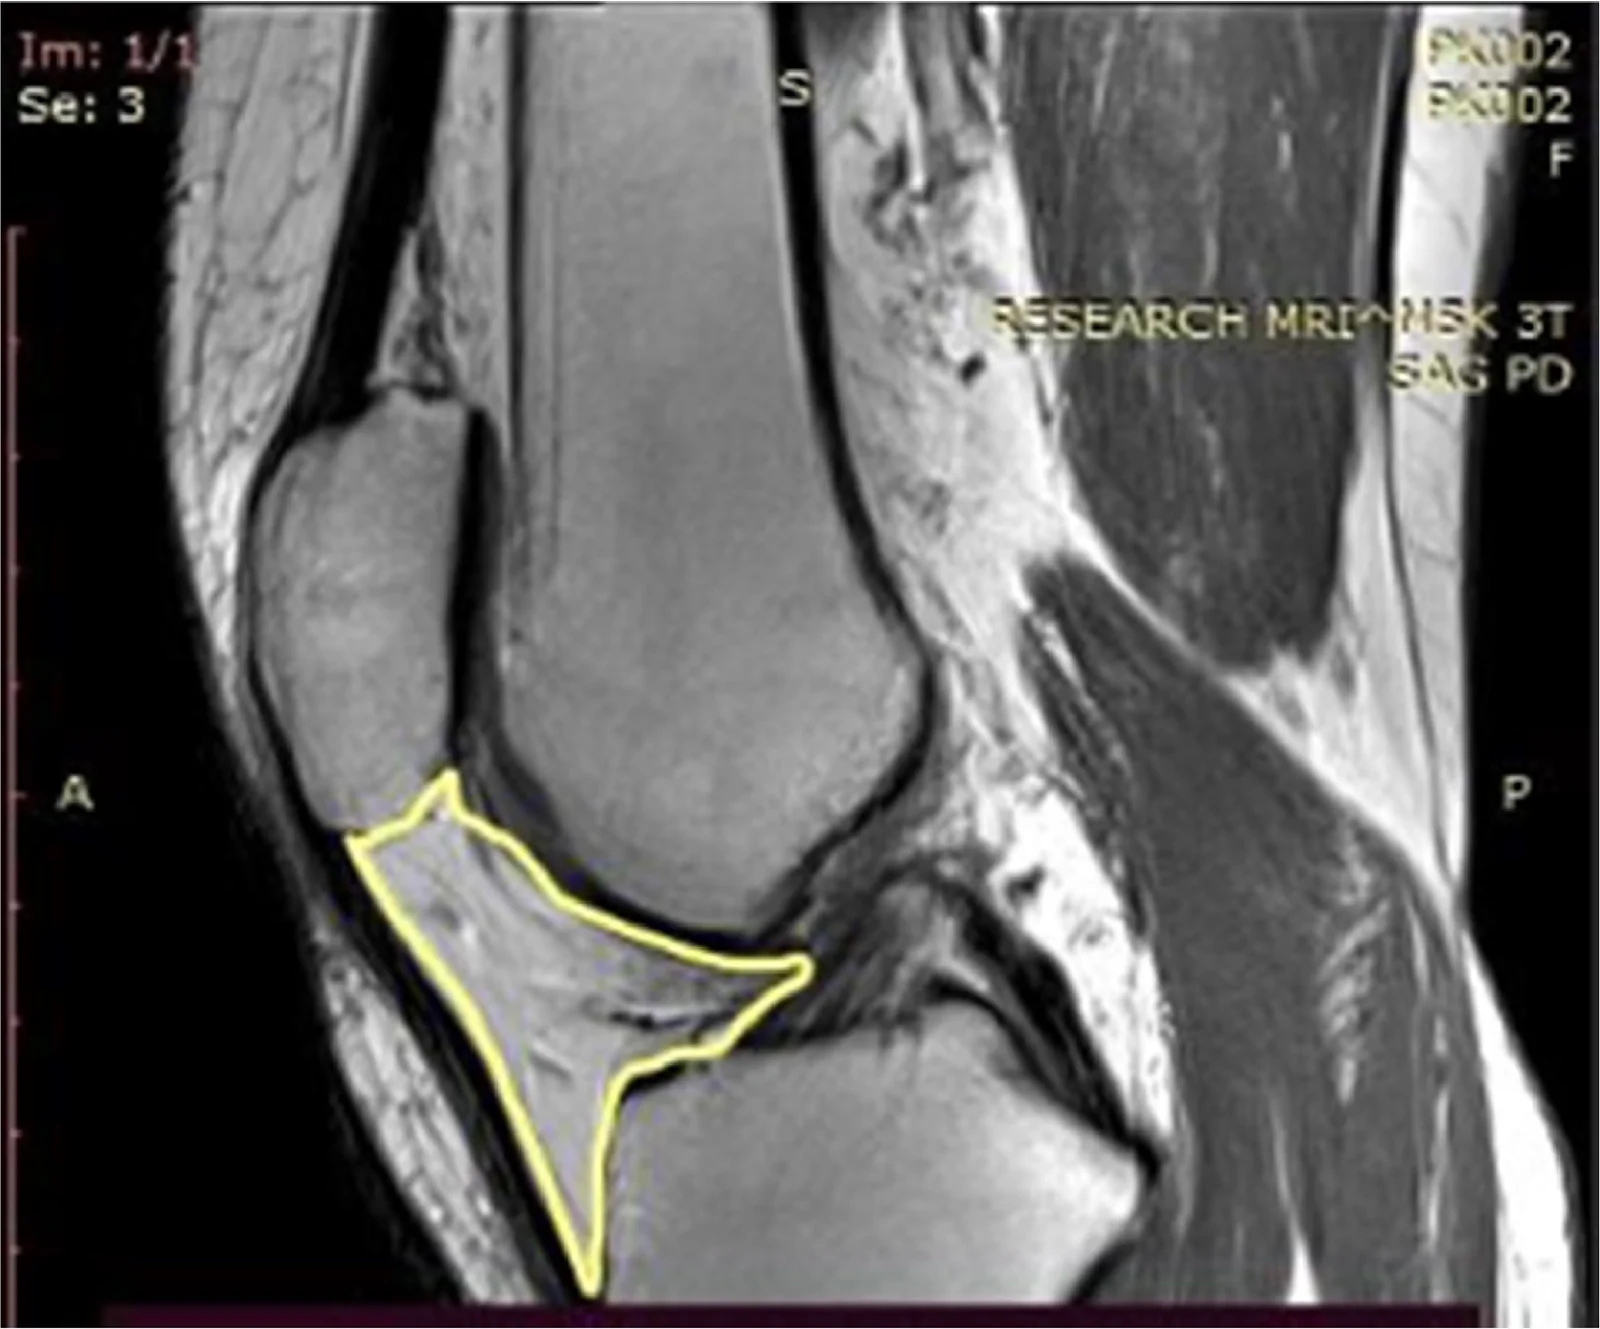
Sample image from a sagittal view PDW MRI demonstrating the IPFP boundaries (yellow).
